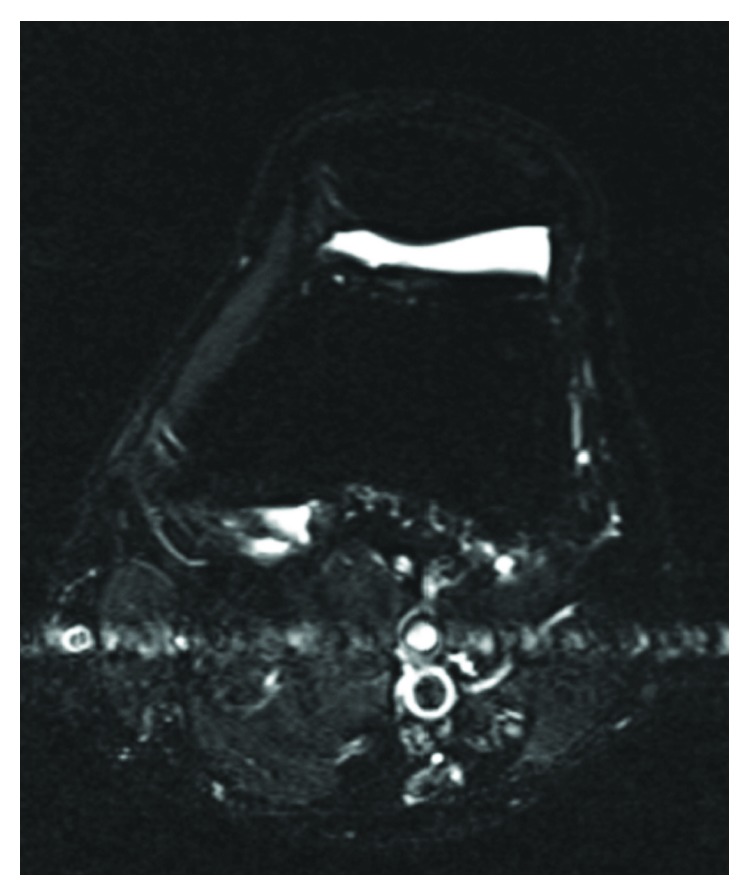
10-month postoperative axial STIR MRI. The fat pad was completely removed and no recurrences were found.
